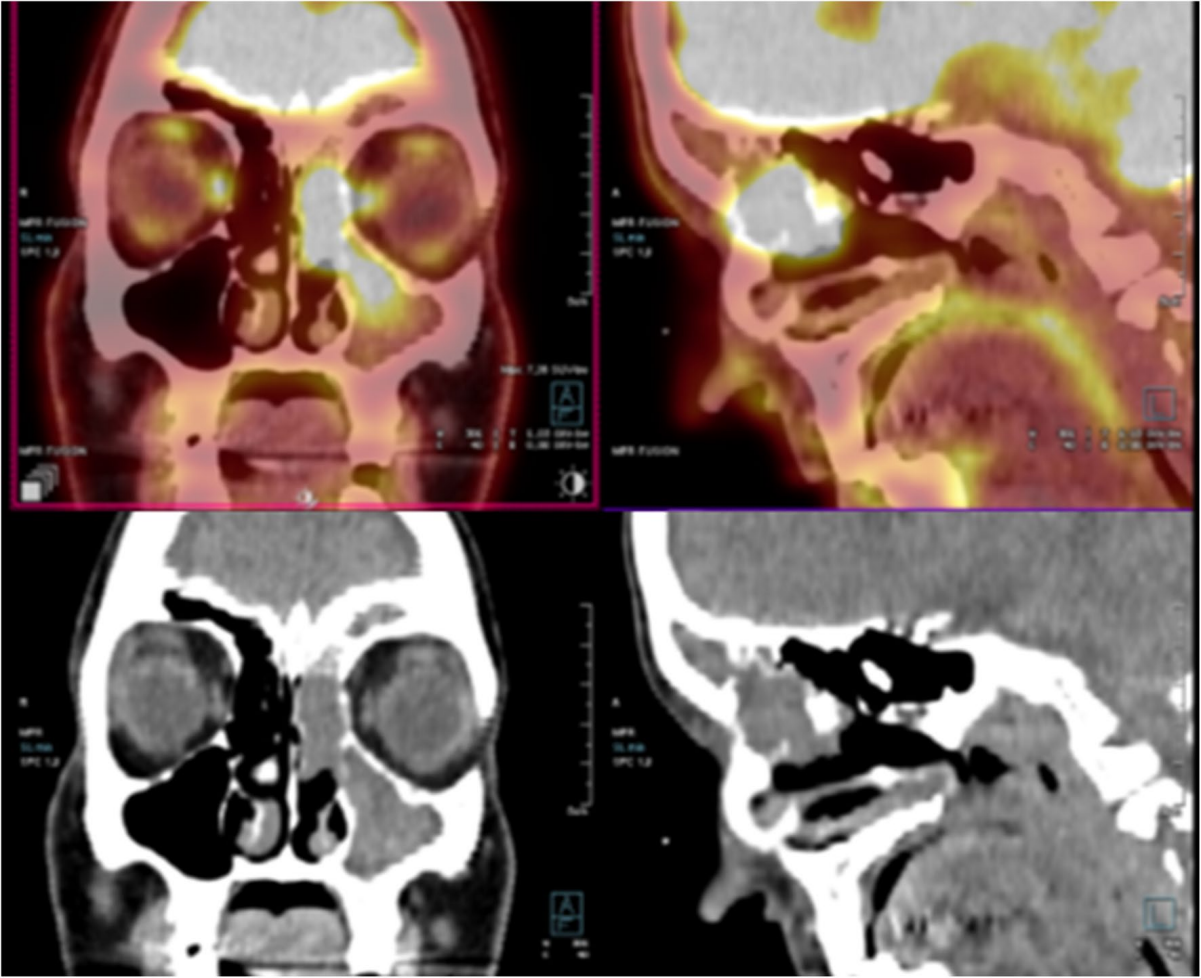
PET/CT imaging study performed in case 1. The tumor filled the left maxillary sinus, extended into the nasal cavity, and infiltrated into the left orbit
